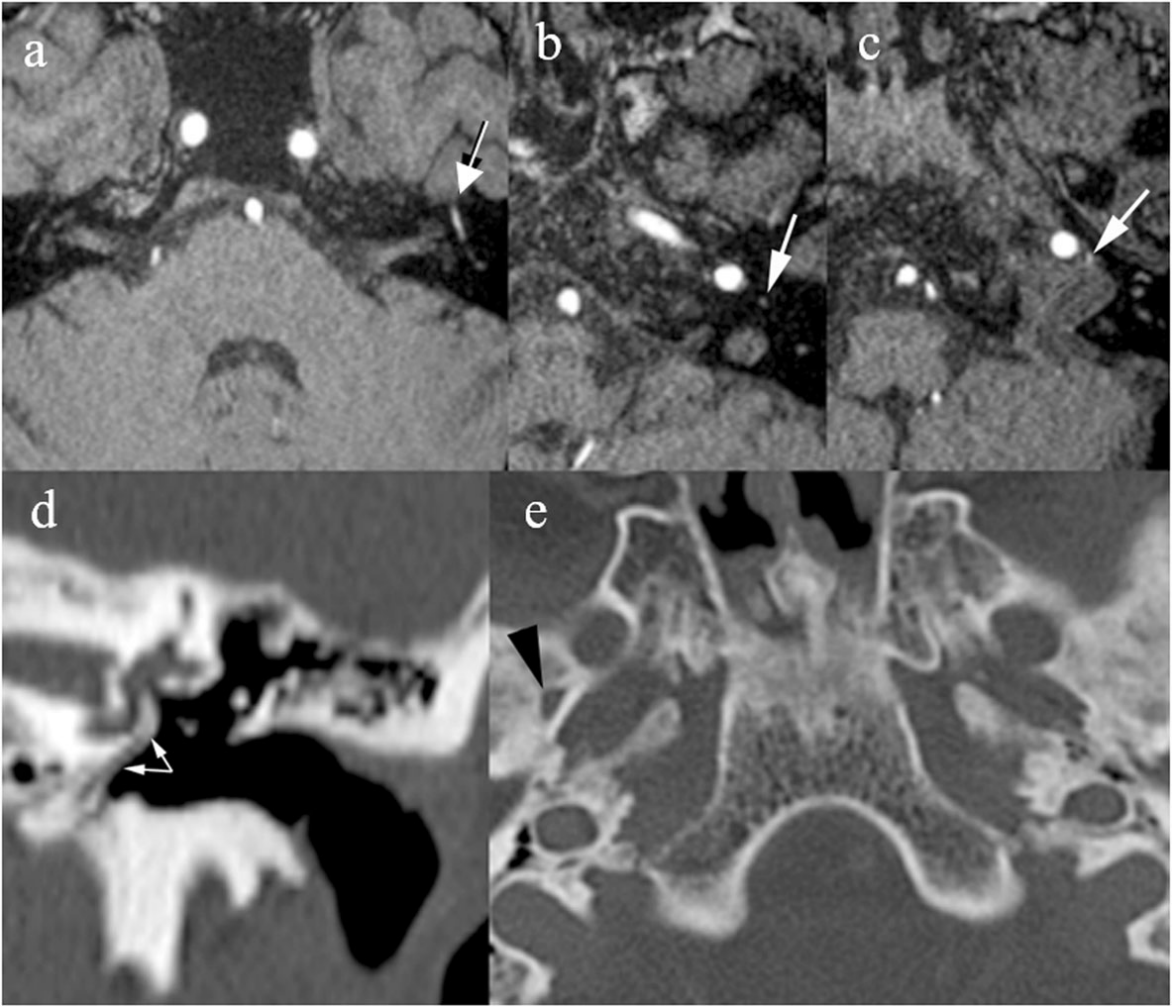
Persistent stapedial artery. Axial partitions, TOF sequence with selective venous flow suppression (a–c). A thin arterial structure (arrows) is seen close to the ICA (c) along the promontory (b) and along the tympanic segment of the facial nerve (a). CT of the brain (d,e): coronal multiplanar reformation (MPR) and native axial acquisition. A linear structure runs along the cochlear promontory (arrows), and the left foramen spinosum is absent (black arrowhead points to the right foramen, normally present). Findings are consistent with a persistent stapedial artery
